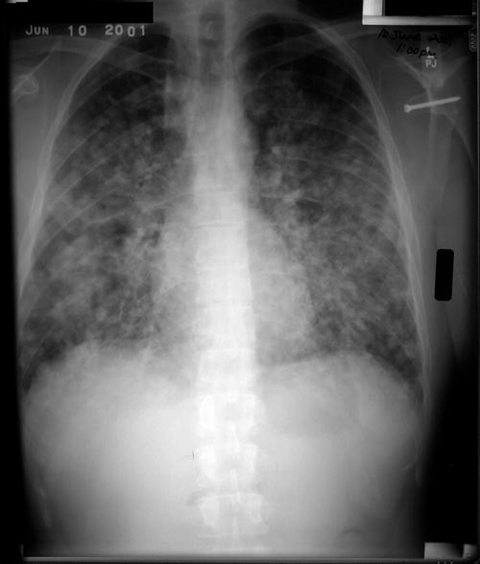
Chest radiograph of patient who acquired acute pulmonary histoplasmosis after visiting a cave in Nicaragua.
